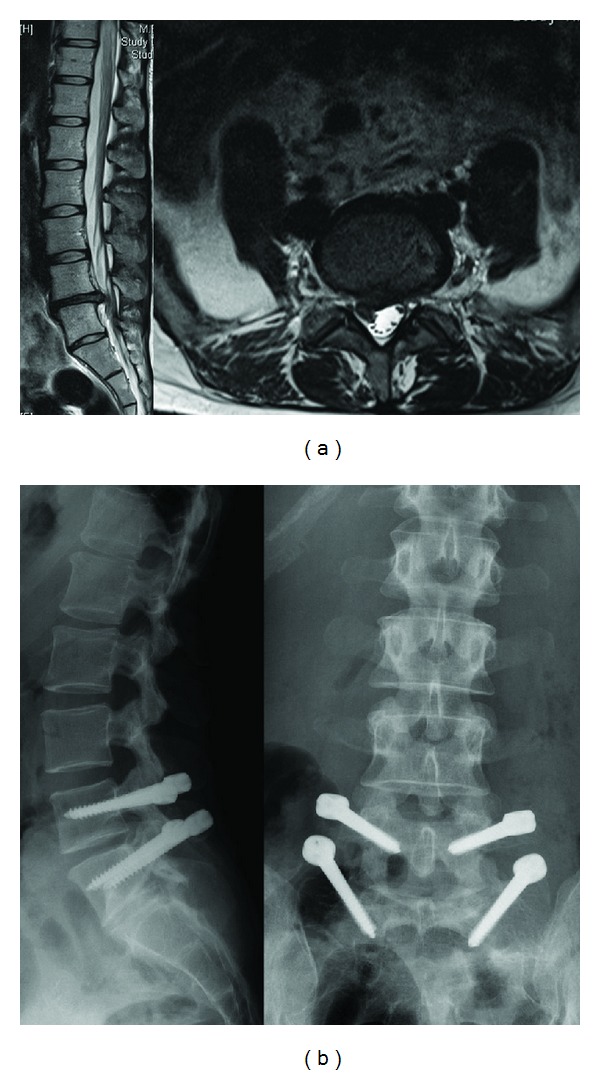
34-year-old female patient with right leg pain and back pain. (a) T2 weighted MRI scans show L4-5 disc herniation; (b) the Dynesys system was applied.
